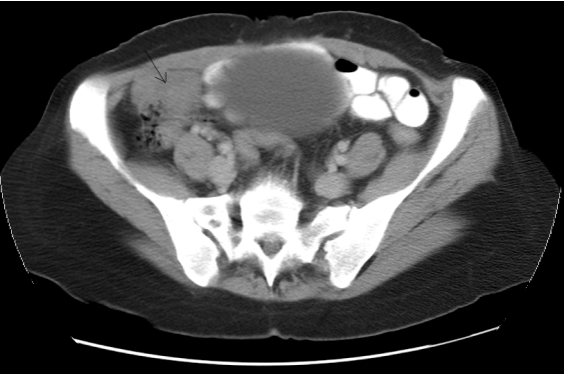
Computed tomography scan showing a mass in the right iliac fossa region.
